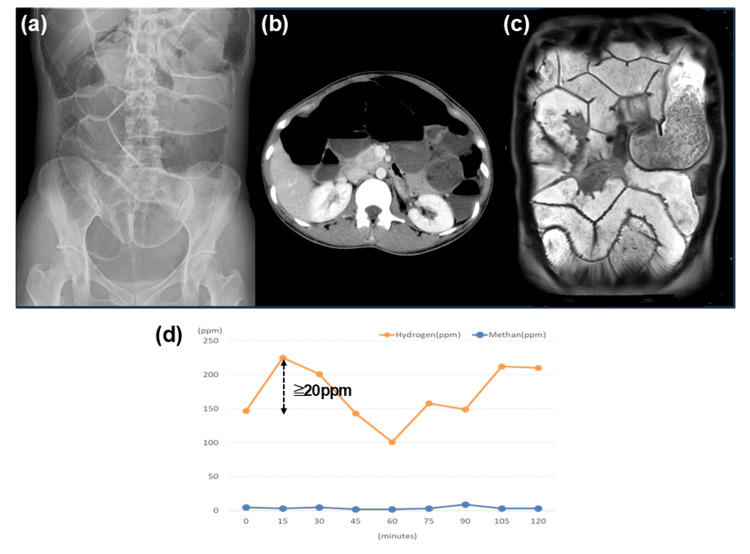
Radiologic and functional imaging findings in CIPO with hydrogen-positive SIBO (case 5) a Abdominal radiograph: marked distension of the intestine by intestinal gas is observed. Small intestinal gas occupies the largest part. b Abdominal CT: markedly dilated intestinal loops and air-fluid levels are seen. c Cine-MRI: small bowel dilatation and reduced peristalsis are seen. d: A breath test confirmed the diagnosis of hydrogen-type SIBO according to the North American Consensus. SIBO: small intestinal bacterial overgrowth; CIPO: chronic intestinal pseudo-obstruction; CT: computed tomography; MRI: magnetic resonance imaging
